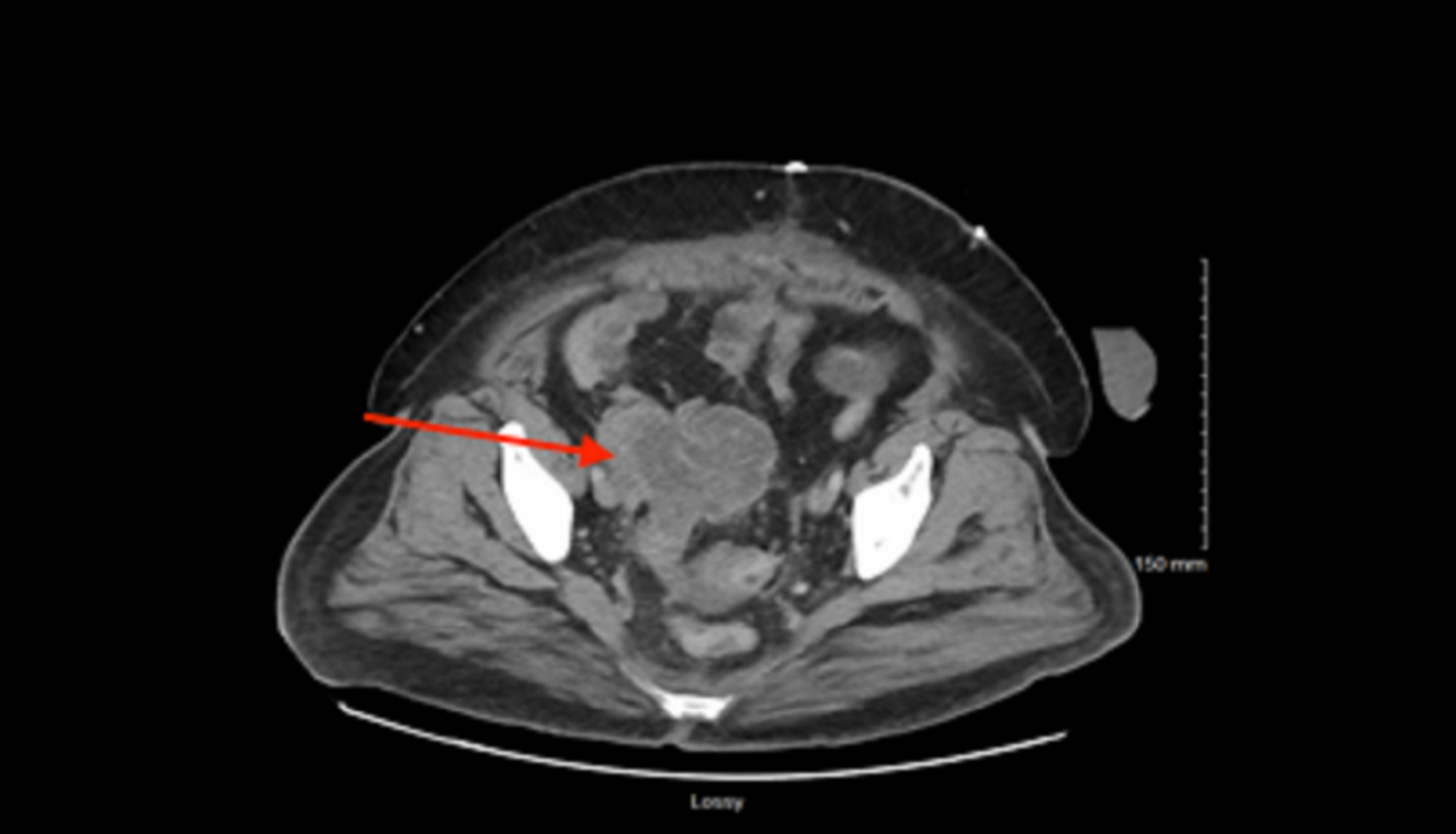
CT of the abdomen and pelvis showing the mass.
